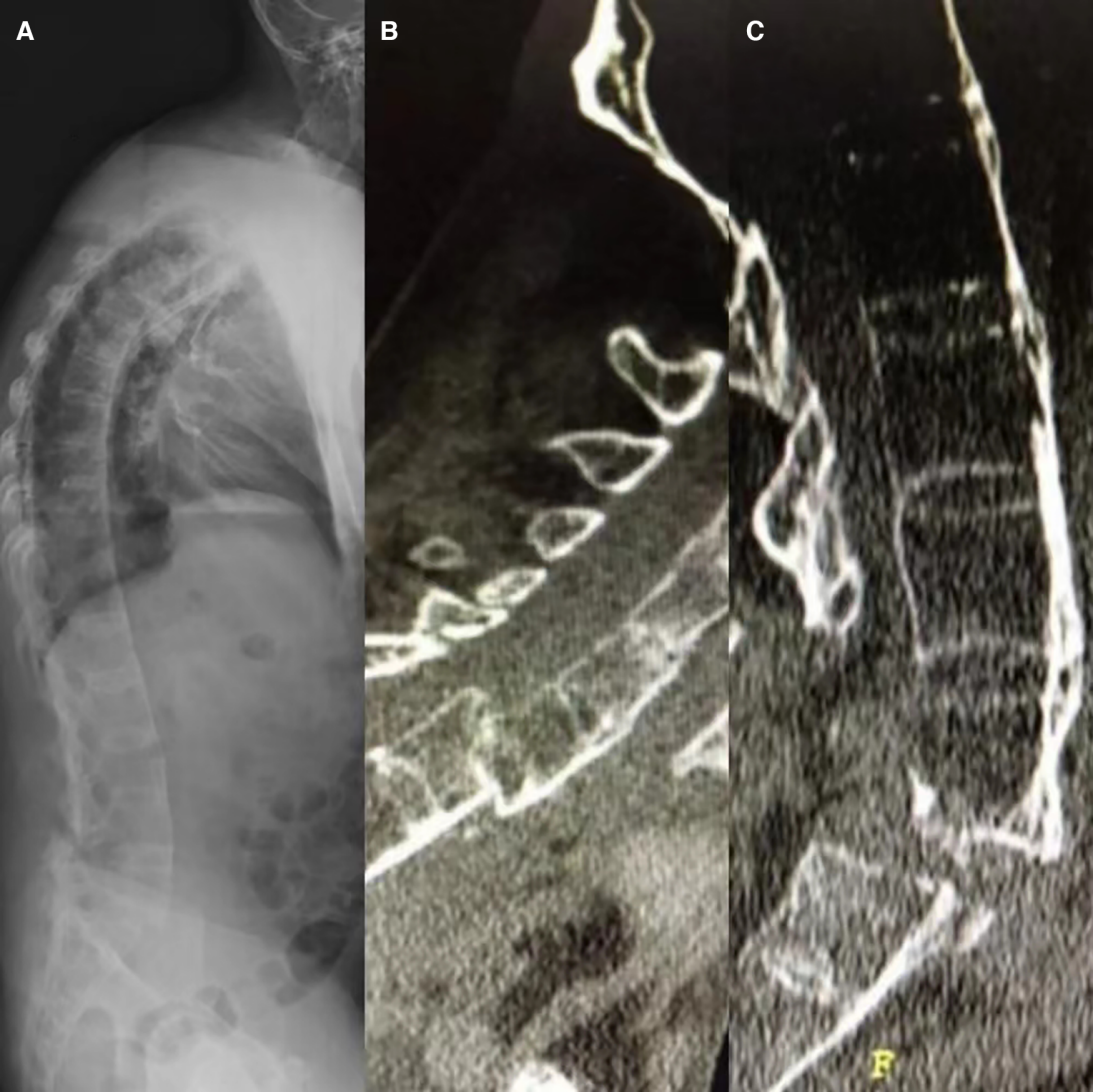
A 57-year-old male with ankylosing spondylitis presented with a lumbar fracture (L4–5). Plain radiographs of the full spine were unremarkable in detecting a cervical spine fracture in this patient (A). A sagittal CT scan also revealed a displaced and unstable fracture–dislocation of C6/7 (B) and L5/S1 (C).
